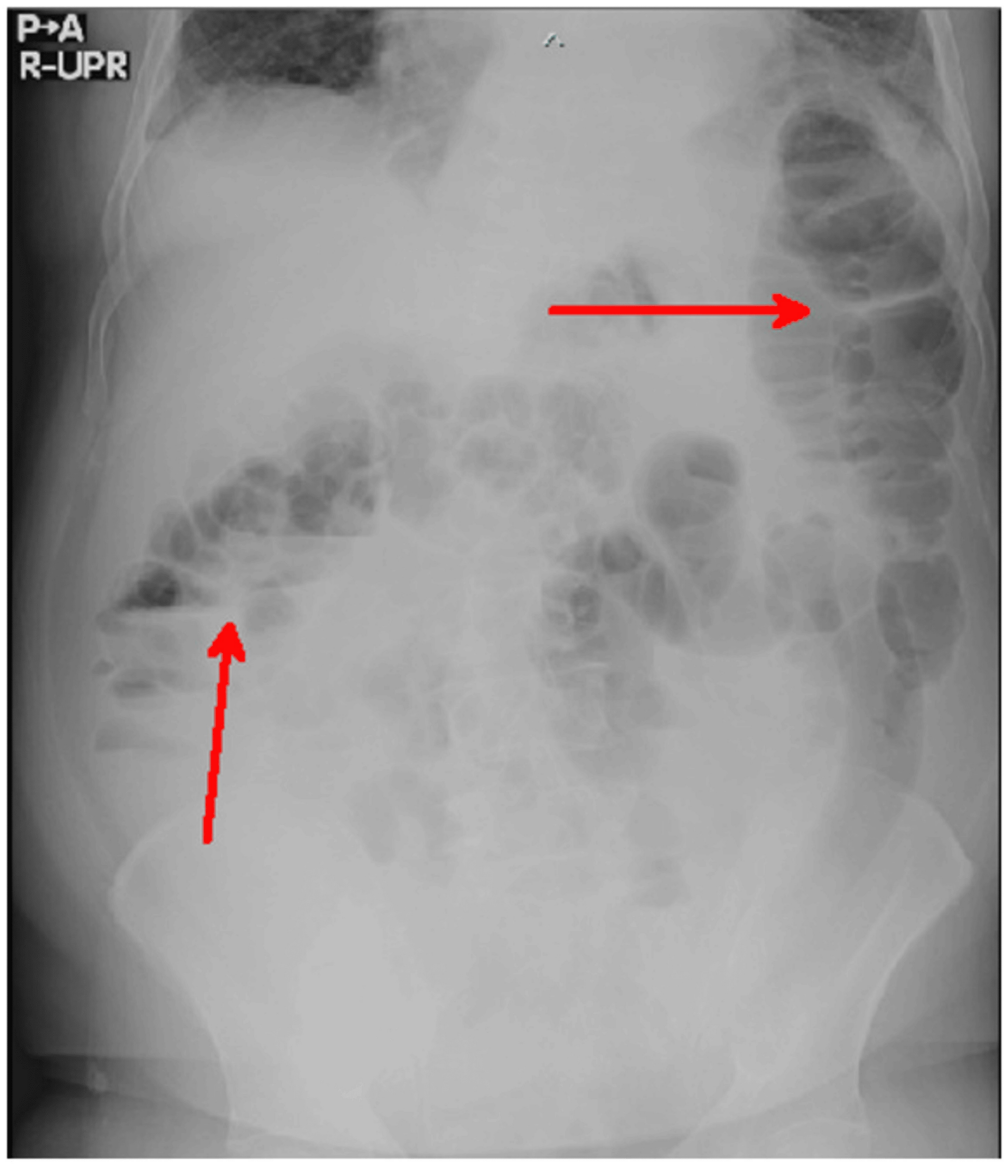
An abdominal erect X-ray The erect abdominal X-ray shows dilation of the small bowel with an air-fluid level on the left side and a similar finding with a distended section of the colon on the right side, as indicated by the arrows.
